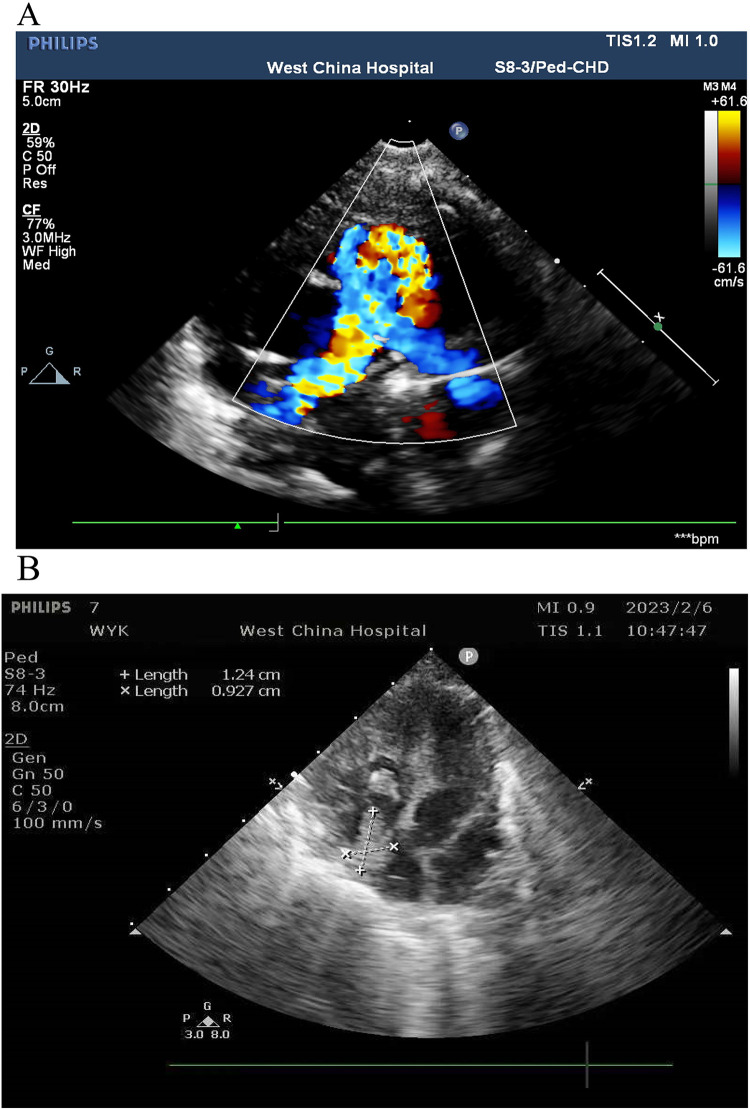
(A) Preoperative cardiac ultrasound. (B) Postoperative cardiac ultrasound.
